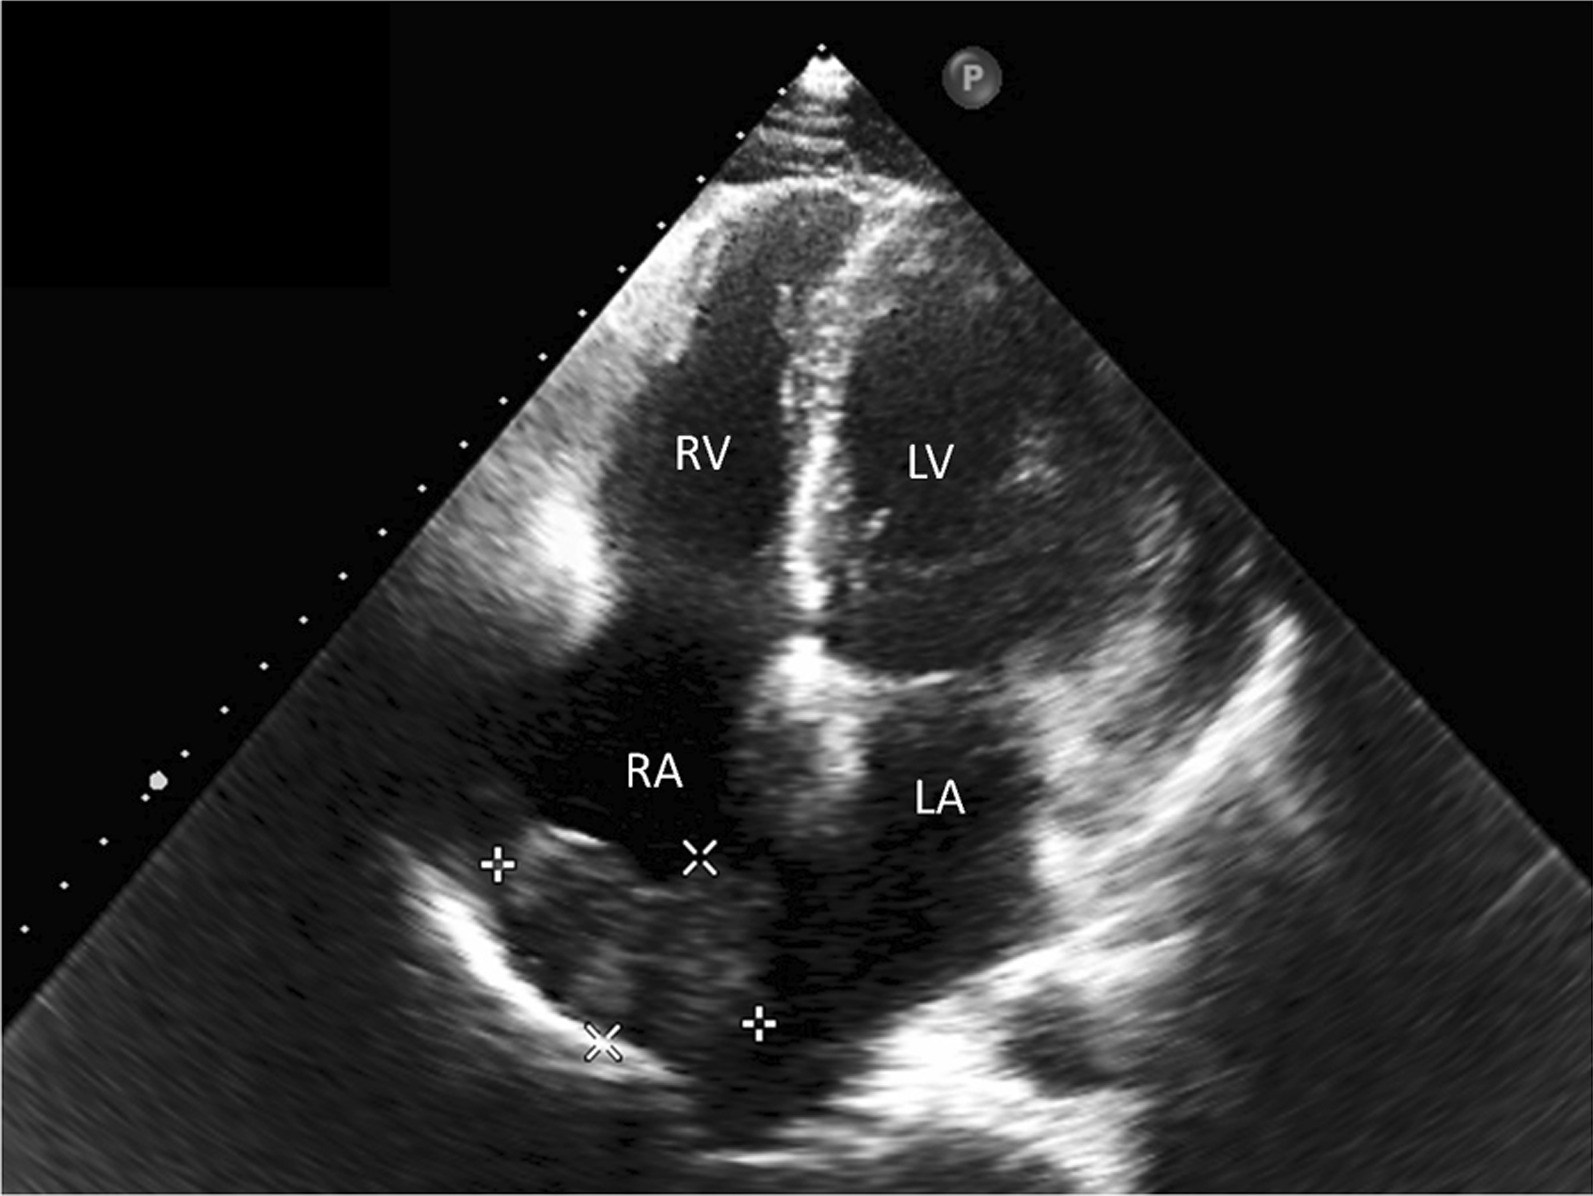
The echocardiogram revealed the presence of a moderately echogenic mass (white crosses) within the right atrium. The mass measures approximately 53 × 30 mm in size with an irregular morphology and relatively well-defined margins, displaying relatively uniform internal echo. *RV* Right ventricle, *LA* Left atrium, *RA* Right atrium, *LV* Left ventricle
